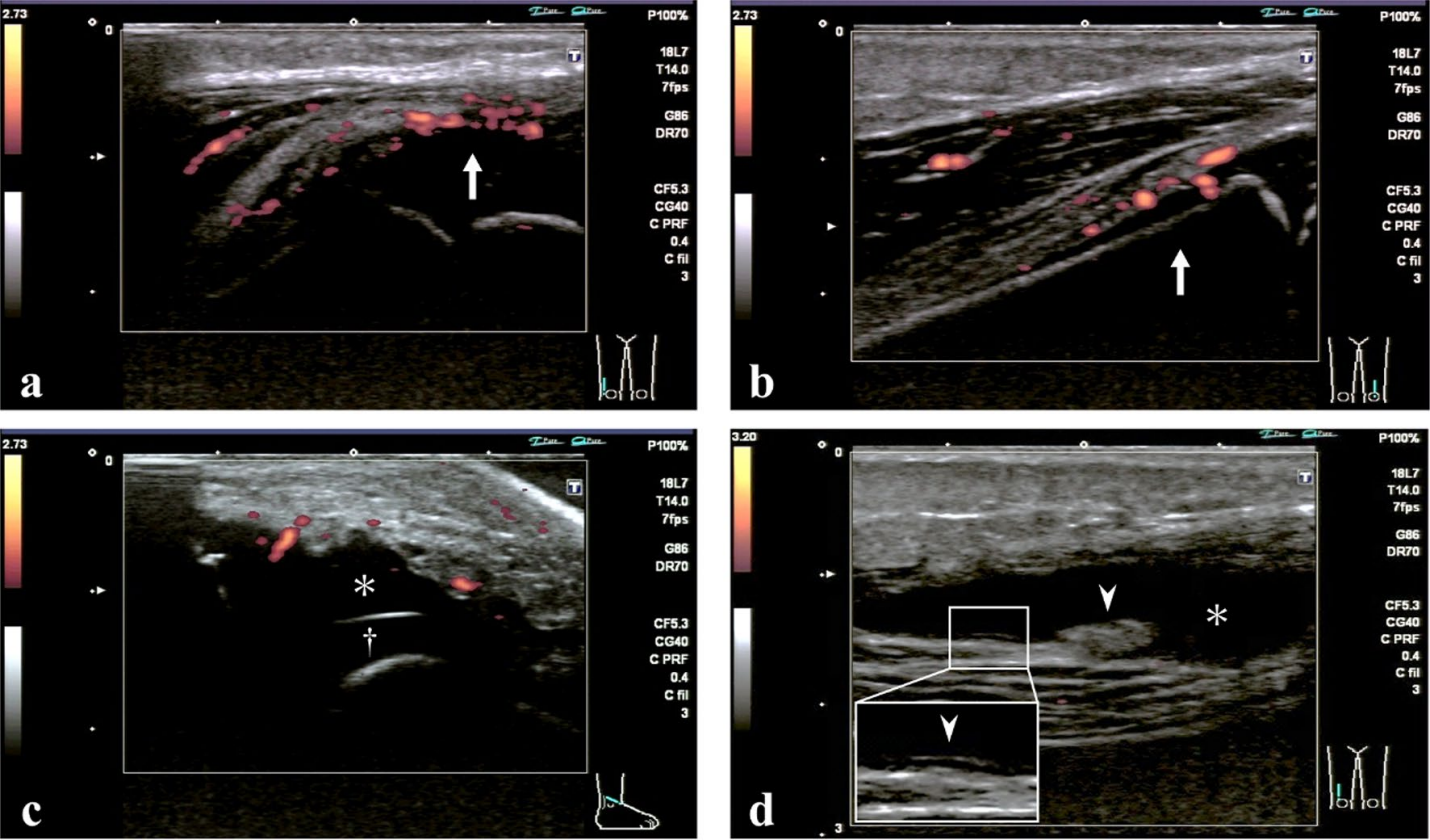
Representative musculoskeletal ultrasonography images. (a, b) Images from a boy aged 2 years. The knees were examined bilaterally revealing PD signals (arrow) on the periarticular soft tissue. (c) Image from a boy aged 1 year. Increased joint fluid (*) was observed on the cartilage (†) in the right ankle. (d) Image from a boy aged 7 years. Increased joint fluid (*) was observed in the right knee, as was linear/nodular edematous thickening of the synovium (arrowhead).
